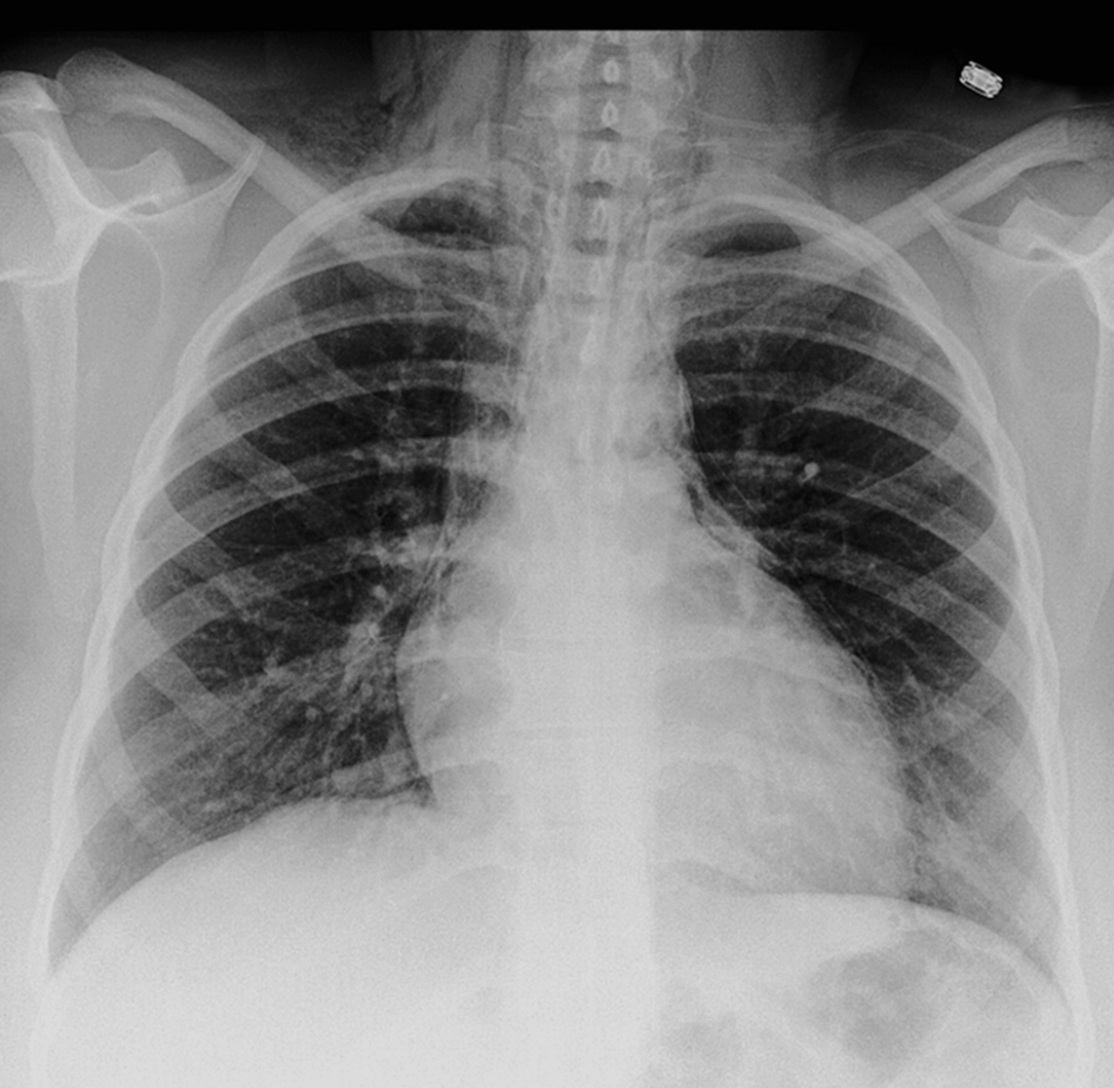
Posteroanterior chest radiograph demonstrating pneumomediastinum, evidenced by thin streaks of air outlining the cardiac silhouette and superior mediastinal structures
